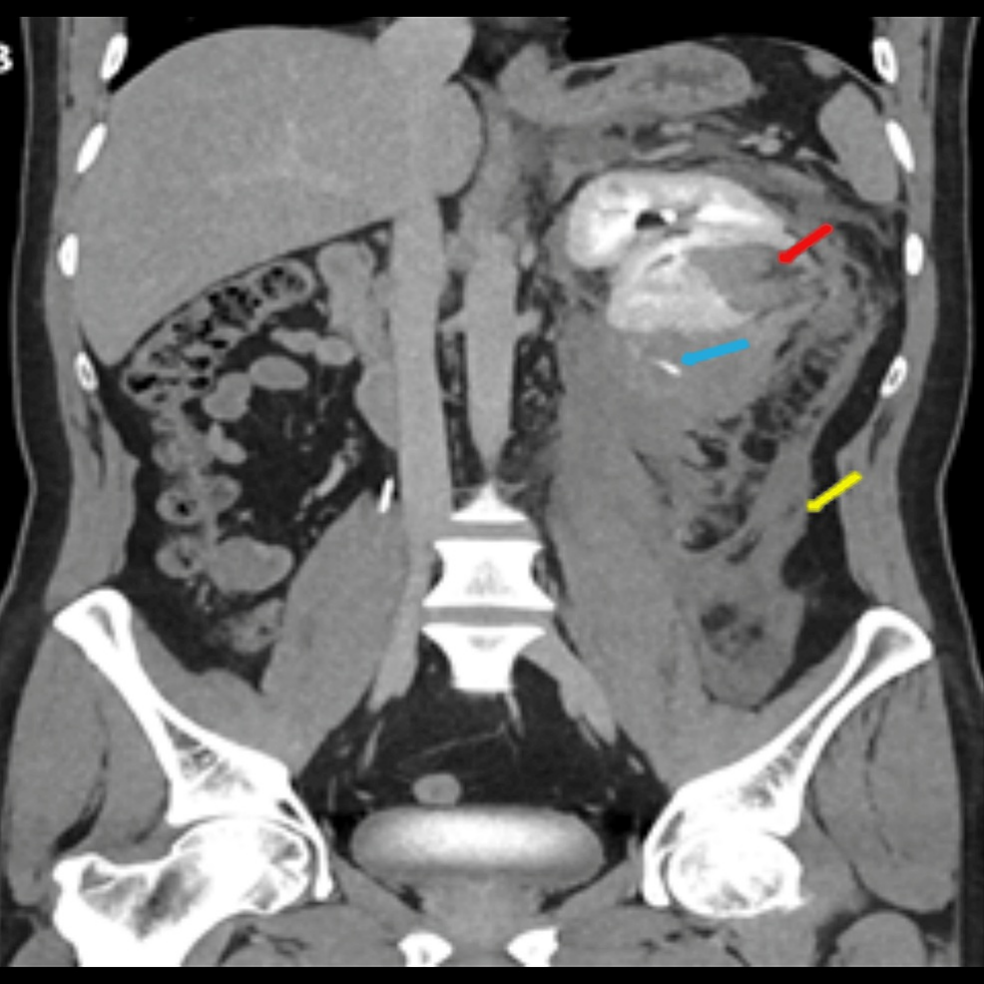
CT abdomen with contrast: coronal cuts showing a large left perinephric hematoma extending inferiorly in the retroperitoneum, reaching the left iliac fossa (yellow arrows). An ill-defined partially exophytic heterogeneously enhancing lesion was noted at the interpolar area of the left kidney (red arrows) with a prominent vessel at the periphery of the lesion, suspicious of minimal contrast extravasation (blue arrow).
